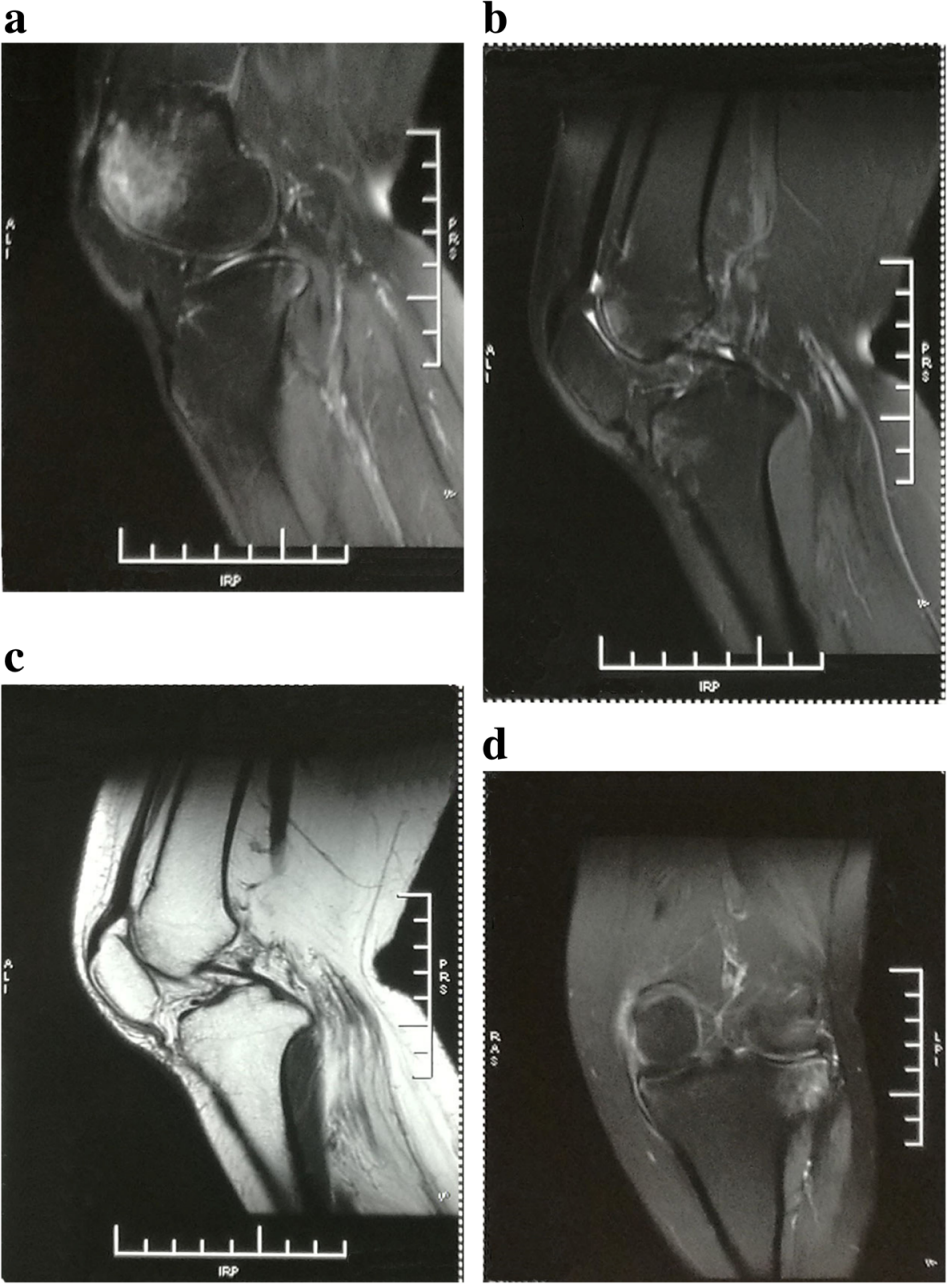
Left knee joint magnetic resonance imaging. It revealed left knee joint injury. a Osteoedema of distal femur; b Rupture of anterior cruciate ligament; c Relaxation of quadriceps femoris tendon and patellar ligament; d Posterolateral osteoedema of tibial plateau
